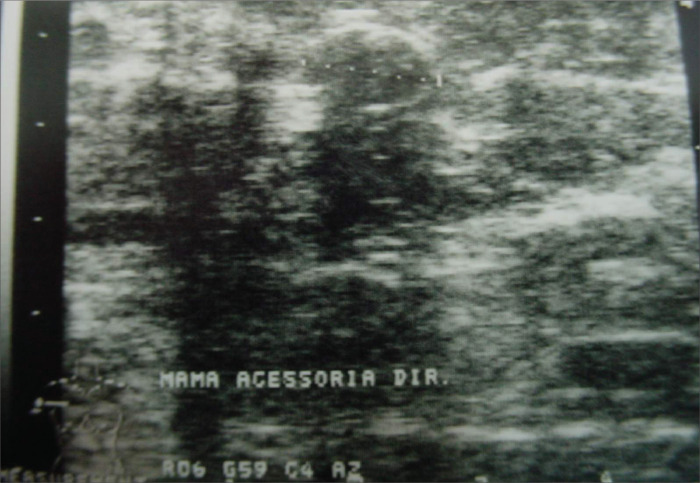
Ultrasound scan of the right axillary supernumerary breast of a 39-year-old woman showing solid, clearly circumscribed homogeneous mass of 1.1 cm diameter.
